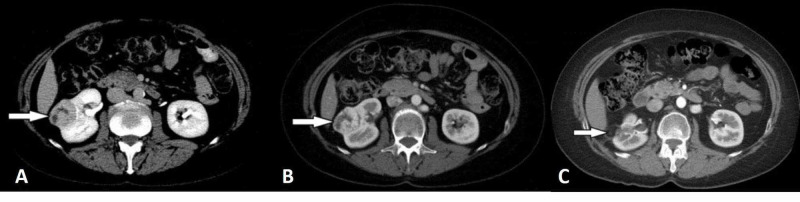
Clear cell renal cell carcinoma (arrows) Computed tomography images taken in the corticomedullary (a) and excretory (b) phase images show typical hypervascularity and subsequent washout of the tumor. In the same patient after nephron-sparing surgery (c), no residual or recurrent tumor is detected.
